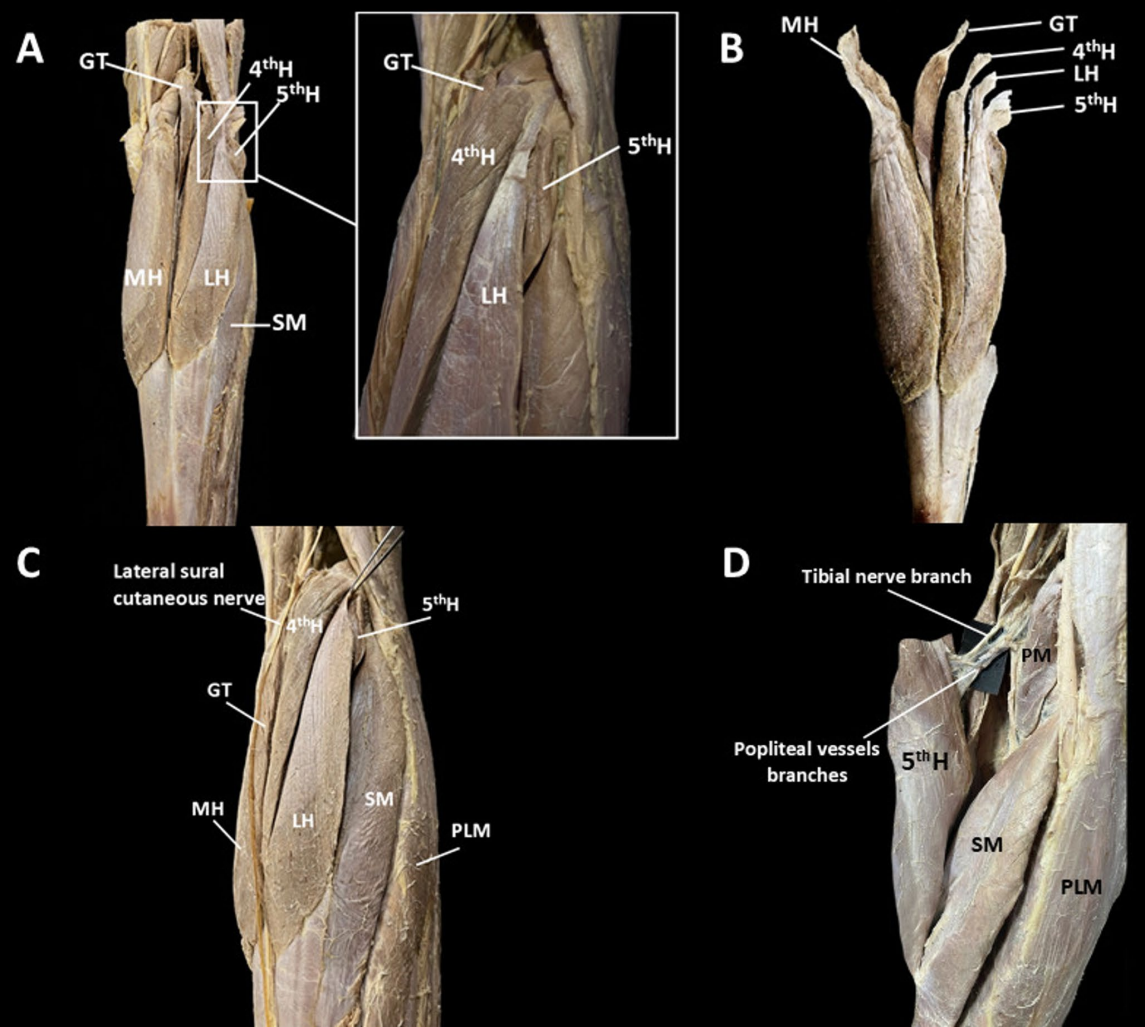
Posterior view of the leg limbs highlighting the right gastrocnemius muscle (GM). A Posterior view of the right leg showing the five heads of the GM. B Dissected GM showing the distinct proximal attachments of its five heads. C Posterolateral view of the five-headed GM showing the lateral sural cutaneous nerve. D Posterior view of the fifth head and its neurovascular bundle. CT, calcaneal tendon; GT, gastrocnemius tertius; L, lateral head; MH, medial head; PLM, peroneus longus muscle; PM, plantaris muscle; SM, soleus muscle
